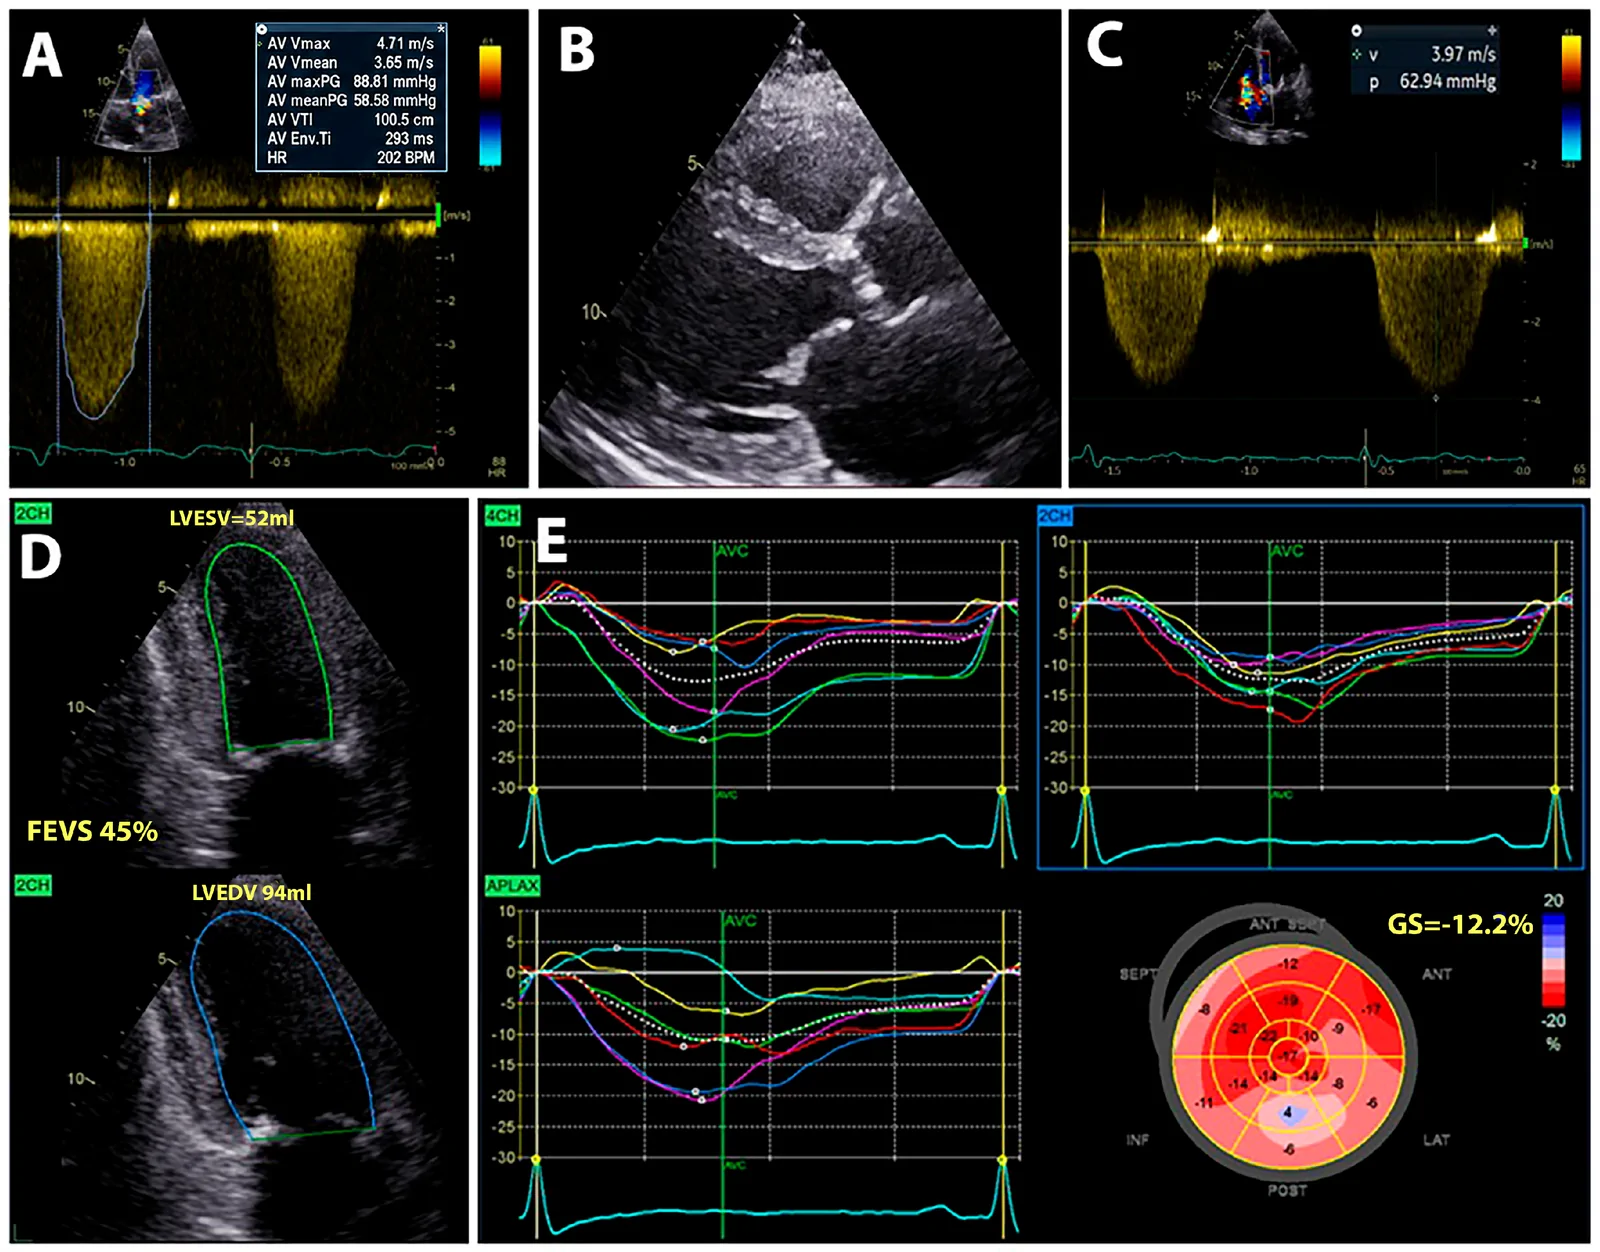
Transthoracic echocardiographic evaluation of aortic valve and cardiac function. (A) Continuous-wave Doppler assessment of aortic valve stenosis. (B) Aortic valve aspect (PLAX view). (C) Severe pulmonary hypertension. (D,E) Myocardial function assessment using LVEF and LV longitudinal strain.
